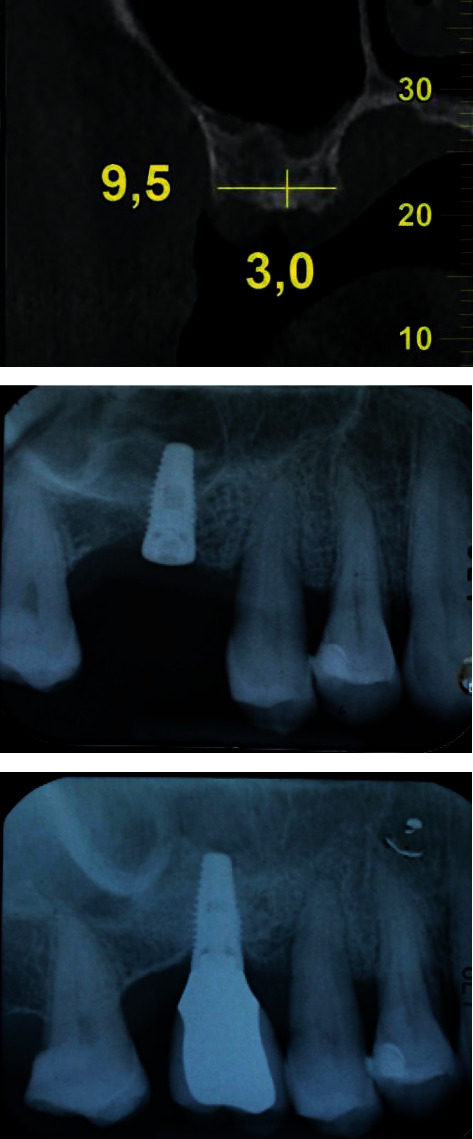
(a) Preoperative CT showing a crestal width of 9.5 mm and a distance of 3 mm from the top of the crest to the lowest point of the maxillary sinus. (b) Periapical radiograph taken immediately after implant insertion. (c) Periapical radiography taken after 9 years of prosthetic loading: note the bone remodeling and the new position of the sinus cortex.
